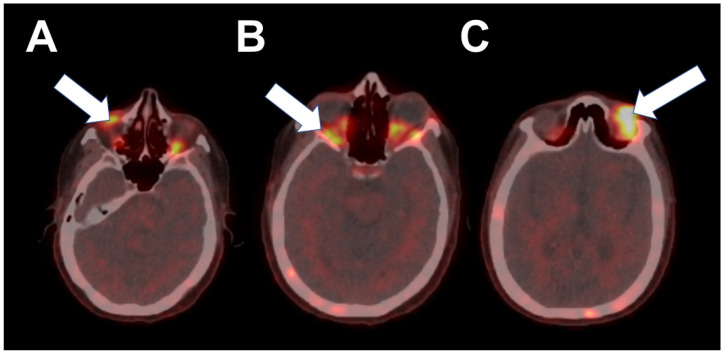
Fused axial PET/CT images demonstrate intense 18F-FES activity in soft tissue in the right inferior (A, arrow) and left superior (C, arrow) orbits and abnormally increased activity in bilateral extraocular muscles (B, arrow).
